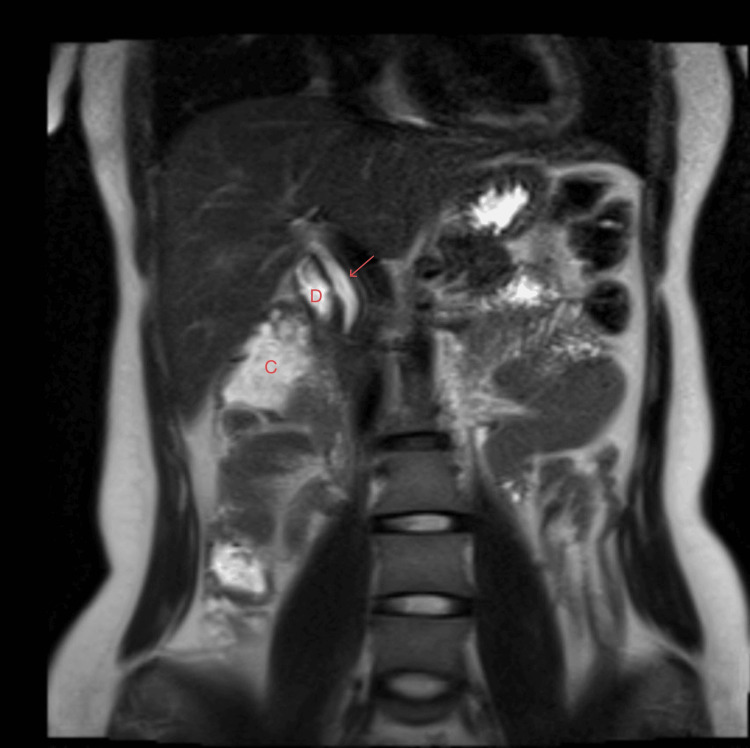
Coronal T2-weighted MRI Coronal T2-weighted MRI of the abdomen showing non-visualization of the gallbladder in the expected anatomical location (arrow, CBD), with adjacent duodenum (D) and colon (C). MRI: magnetic resonance imaging; CBD: common bile duct
